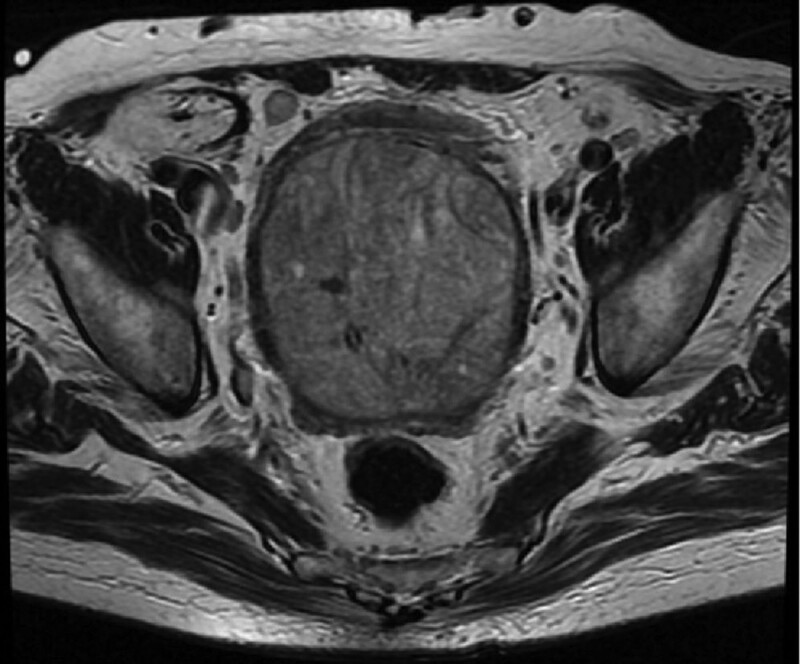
Cross-section of magnetic resonance image, huge prostate filling the pelvic cavity.
